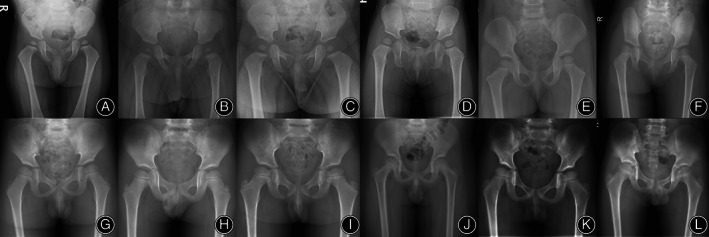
Hip radiographs of children aged from 4 months to 9 years, the femoral neck‐shaft angle decreases with the increase of age. (A): 6 months old; (B): 7 months old; (C): 10 months old; (D): 1 year old; (E): 2 years old; (F): 3 years old; (G): 4 years old; (H): 5 years old; (I): 6 years old; (J): 7 years old. (K): 8 years old; (L): 9 years old.
